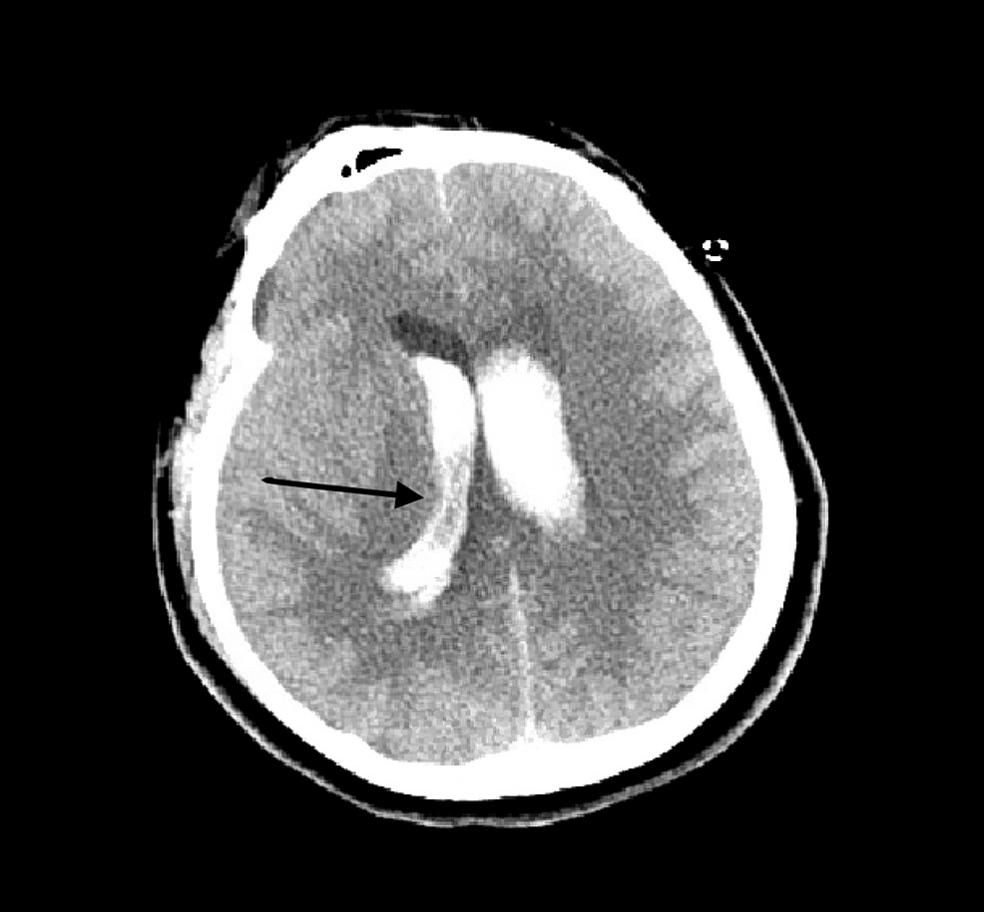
Computed tomography imaging of the brain demonstrating diffuse intraventricular hemorrhage with associated hydrocephalus.
